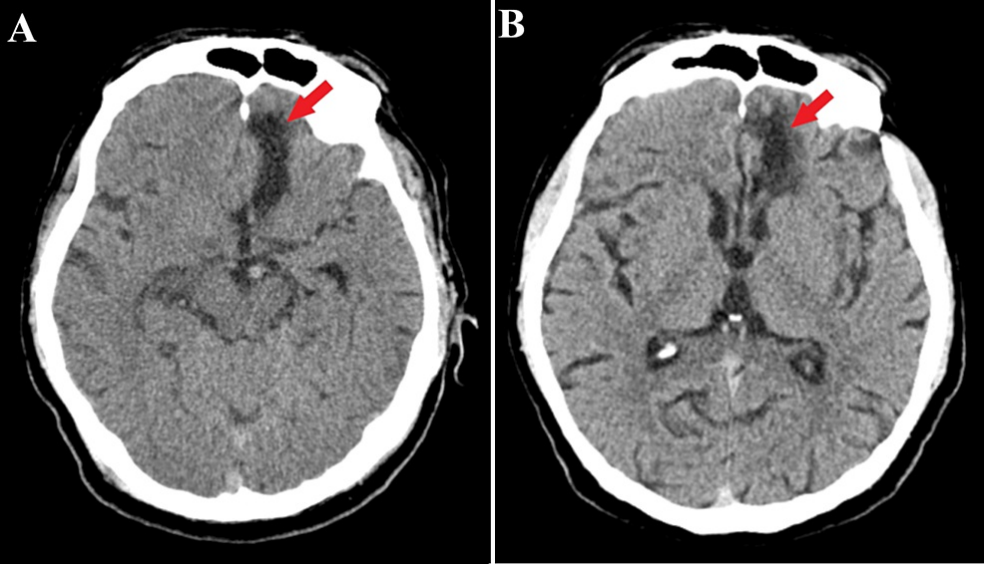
Follow-up CT scans. The axial sections of the CT scan at the four-month follow-up (A) and five-year follow-up (B) highlight a hypodensity area corresponding to the craniectomy (red arrows) and no indications of a recent hemorrhage.
